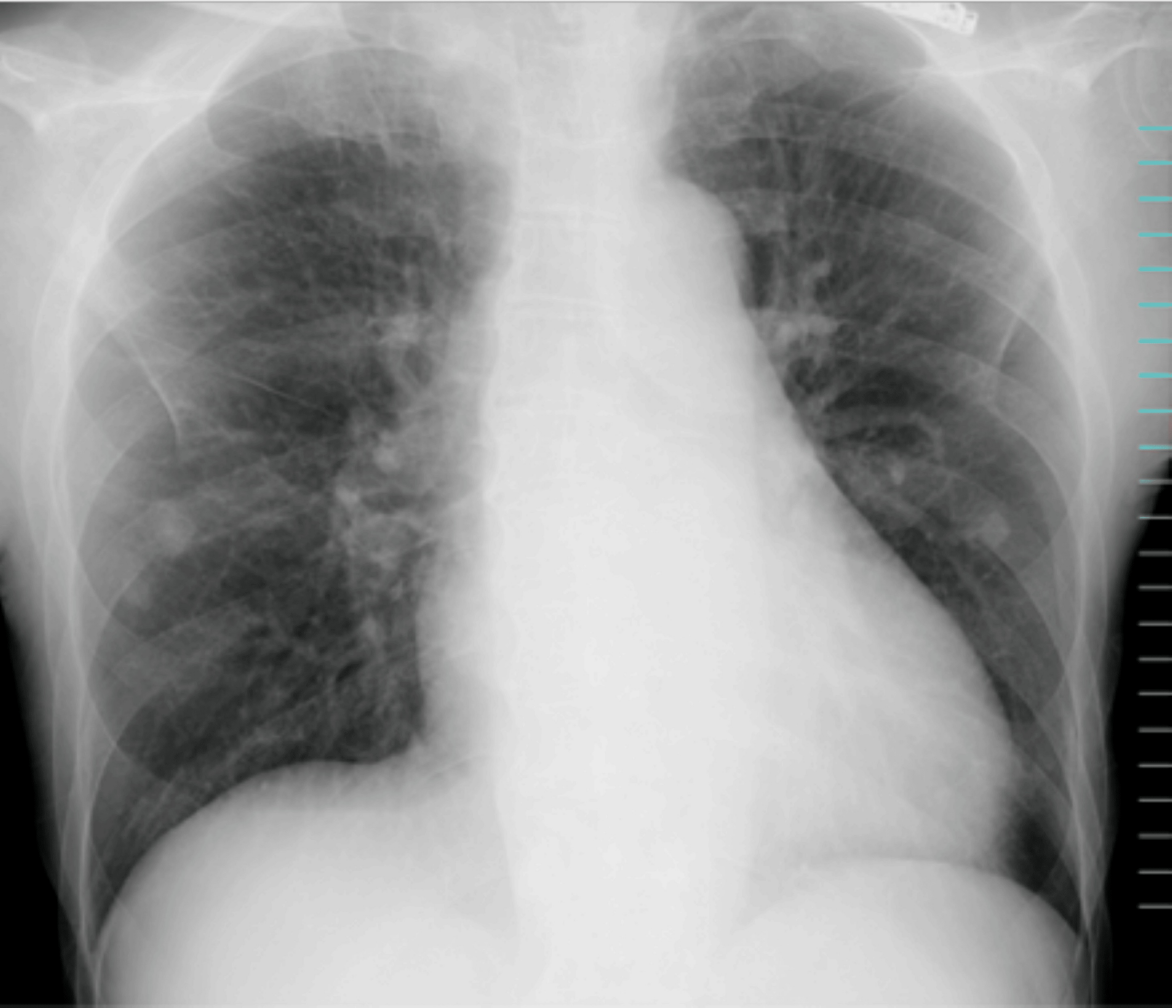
Initial chest X-ray showing clear lung fields and sharp costophrenic angles
